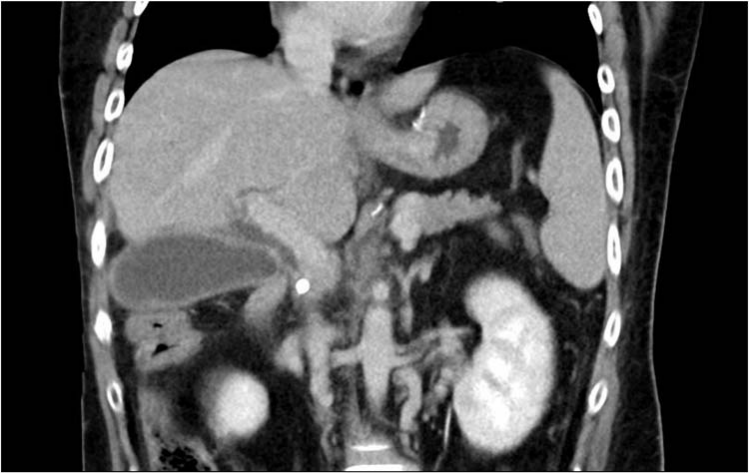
Abdominal CT revealed CBD stone resulting in upstream biliary dilatation and acute cholecystitis.
